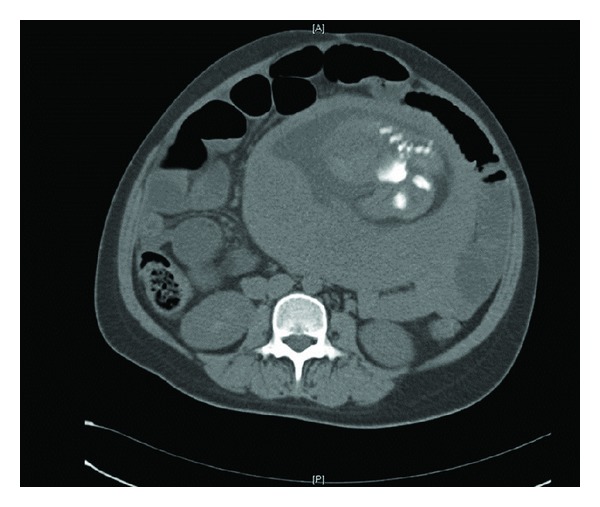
CT scan of patient showing dilated small bowel loops and part of the fetus.
